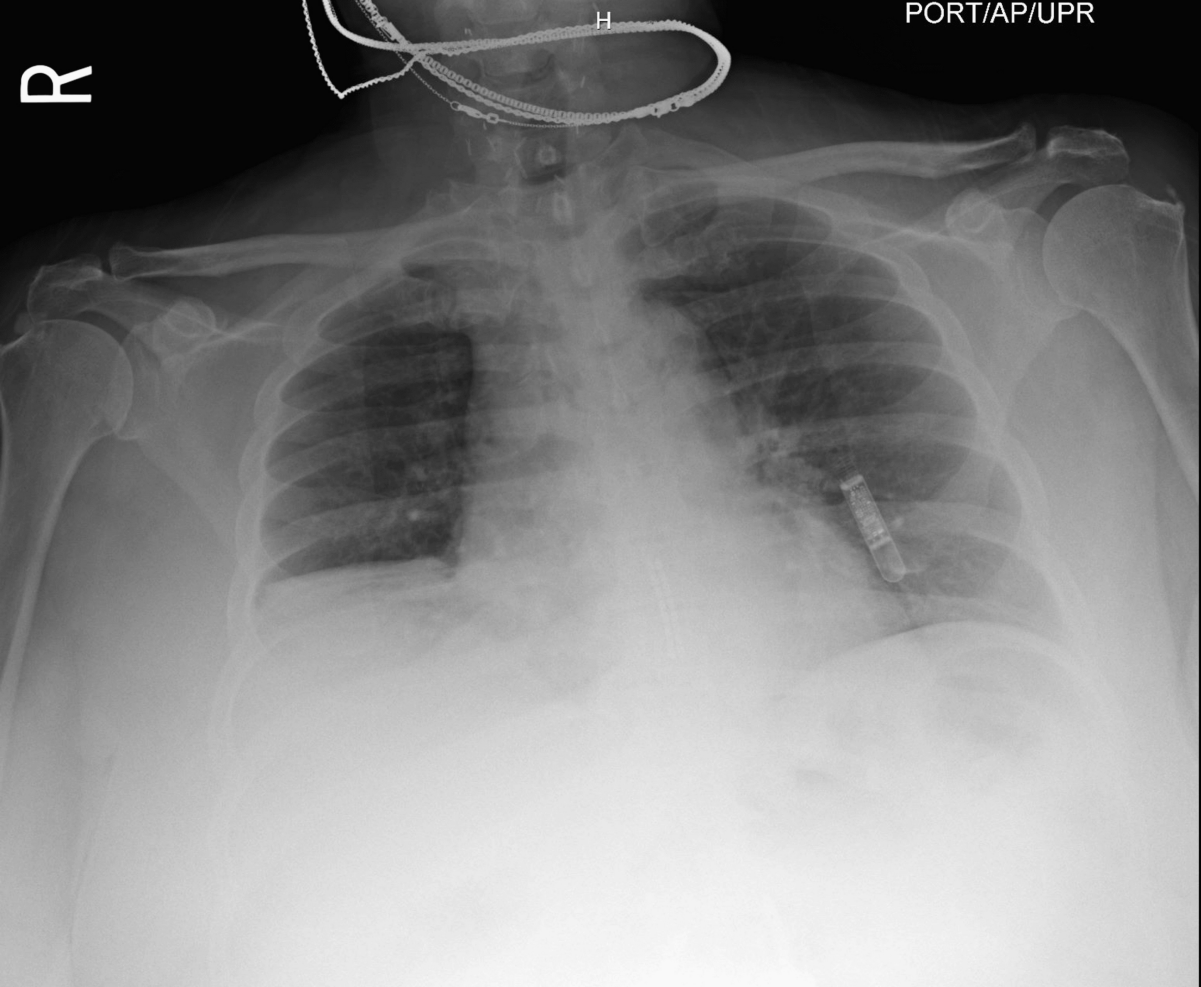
CXR on hospital day 1: no acute cardiopulmonary abnormalities. CXR: chest radiograph
